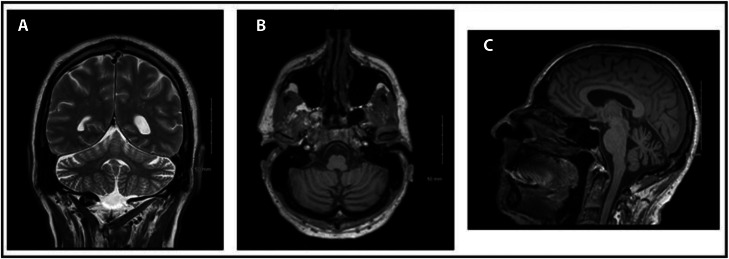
Brain MRI demonstrating cerebellar atrophy. (A) Coronal T2-weighted image showing diffuse cerebellar volume loss with widening of cerebellar folia and fissures. (B) Axial T2-weighted image confirming bilateral cerebellar hemispheric atrophy without focal signal abnormality. (C) Sagittal T1-weighted image demonstrating vermian involvement and enlargement of surrounding cerebrospinal fluid spaces. The absence of a focal lesion favors a degenerative cerebellar process rather than a neoplastic or vascular etiology.
